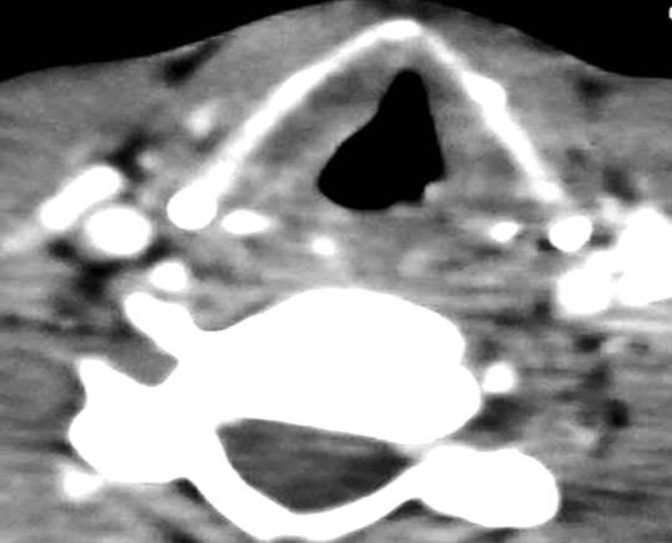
CT scan picture of the glottic growth at left vocal cord
